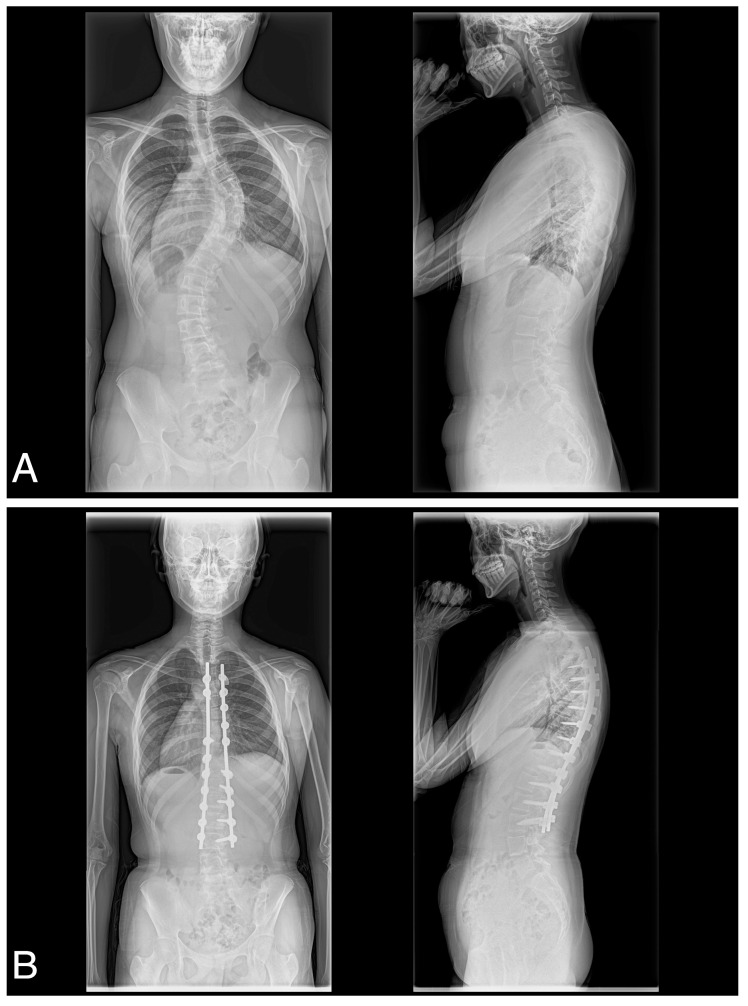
(A) Preoperative posteroanterior and lateral and (B) postoperative posteroanterior and lateral radiographs obtained of a 17-year-old female with Lenke type 1C scoliosis.
